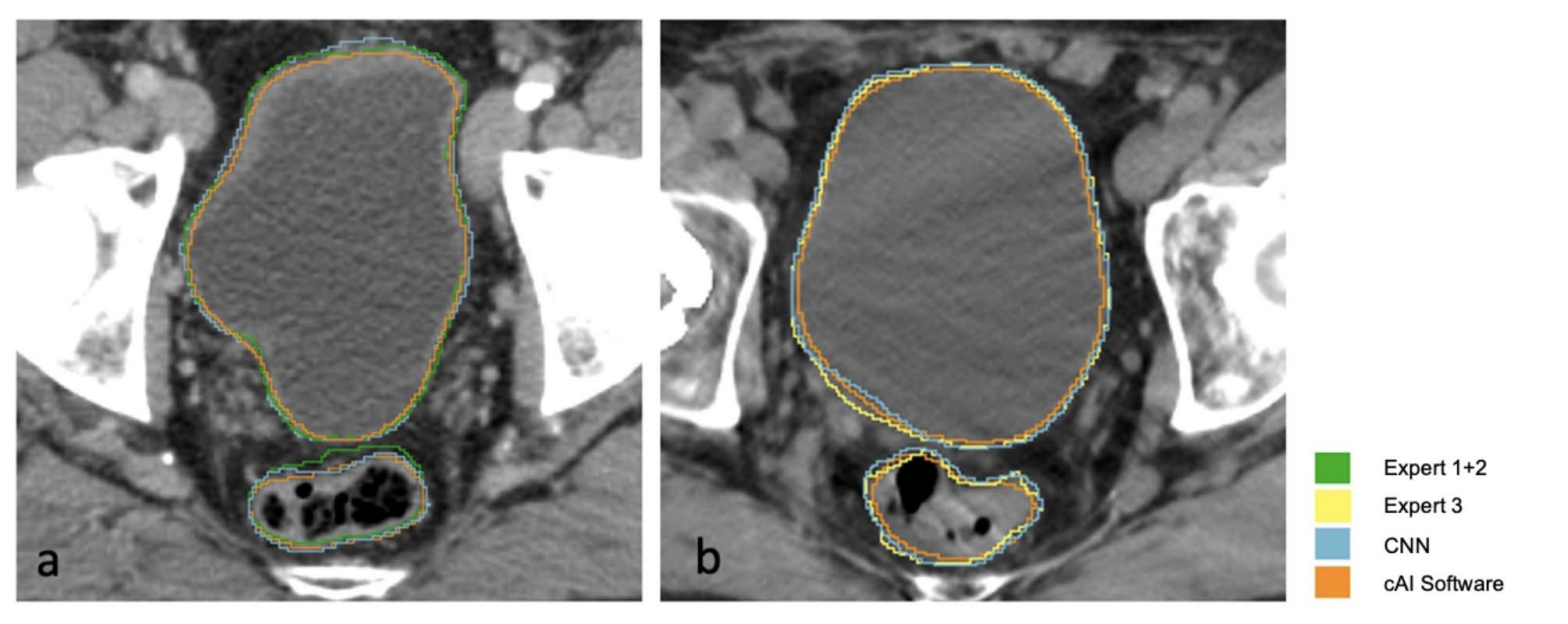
Representative CT datasets from the validation (a) and test cohorts (b). The resulting DSC values for these patients are the same as the median Dice-Sørensen Coefficient (DSC) for the cohorts. Segmentations: expert (green), independent expert (yellow), CNN (blue), cAI (orange).
